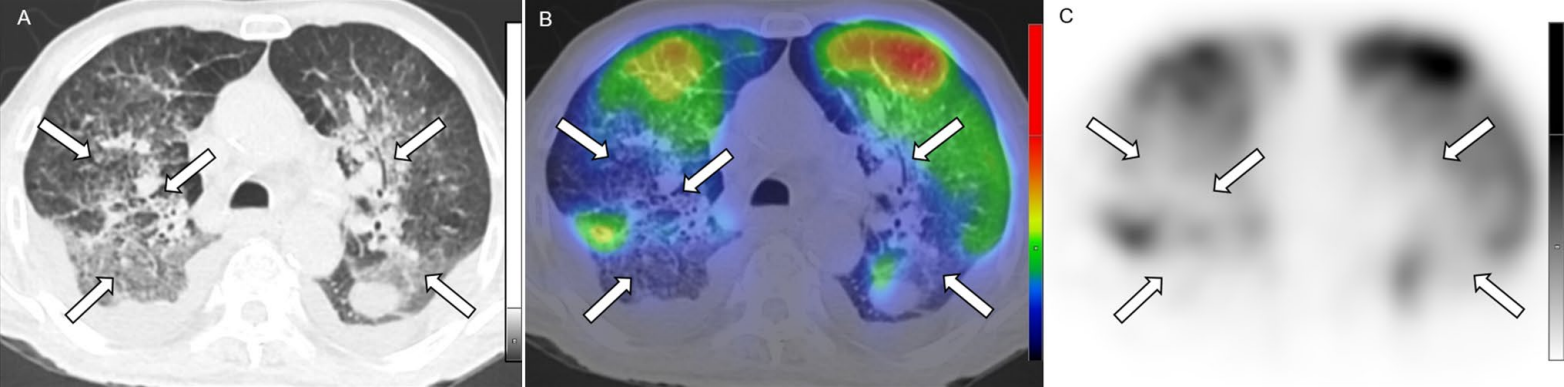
Axial low-dose CT (a), fused Q-SPECT/CT (b), and SPECT images of a COVID-19 patient. Extensive areas with decreased perfusion are detected in both lungs corresponding to ground-glass opacities and consolidations (as demonstrated *white arrows*). Bilateral, pleural effusion with no tracer accumulation could also be visualized in dorsal lung territories. Preserved pulmonary parenchyma shows normal tracer-distribution. *CT* computed tomography, *Q-SPECT/CT* perfusion single-photon emission computed tomography/computed tomography, *COVID-19* coronavirus disease 2019
